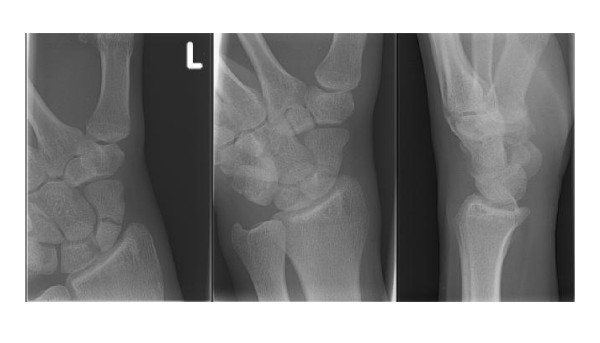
Initial radiograph (patient A): a postero-anterior view; b oblique view; c. lateral view.
